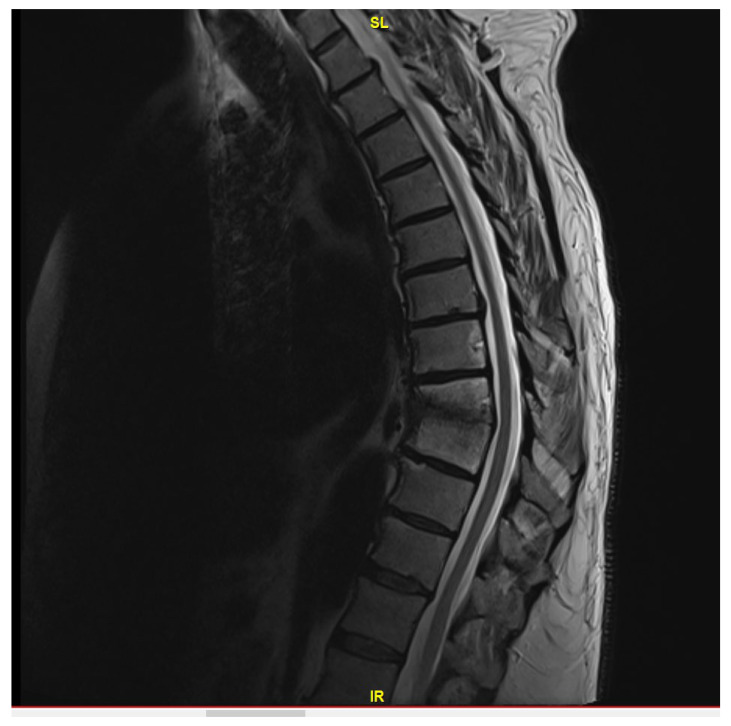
MRI thoracic spine with and without IV contrast revealing redemonstration of T8-T9 diskitis with mild improvement of paraspinal/epidural phlegmon.
